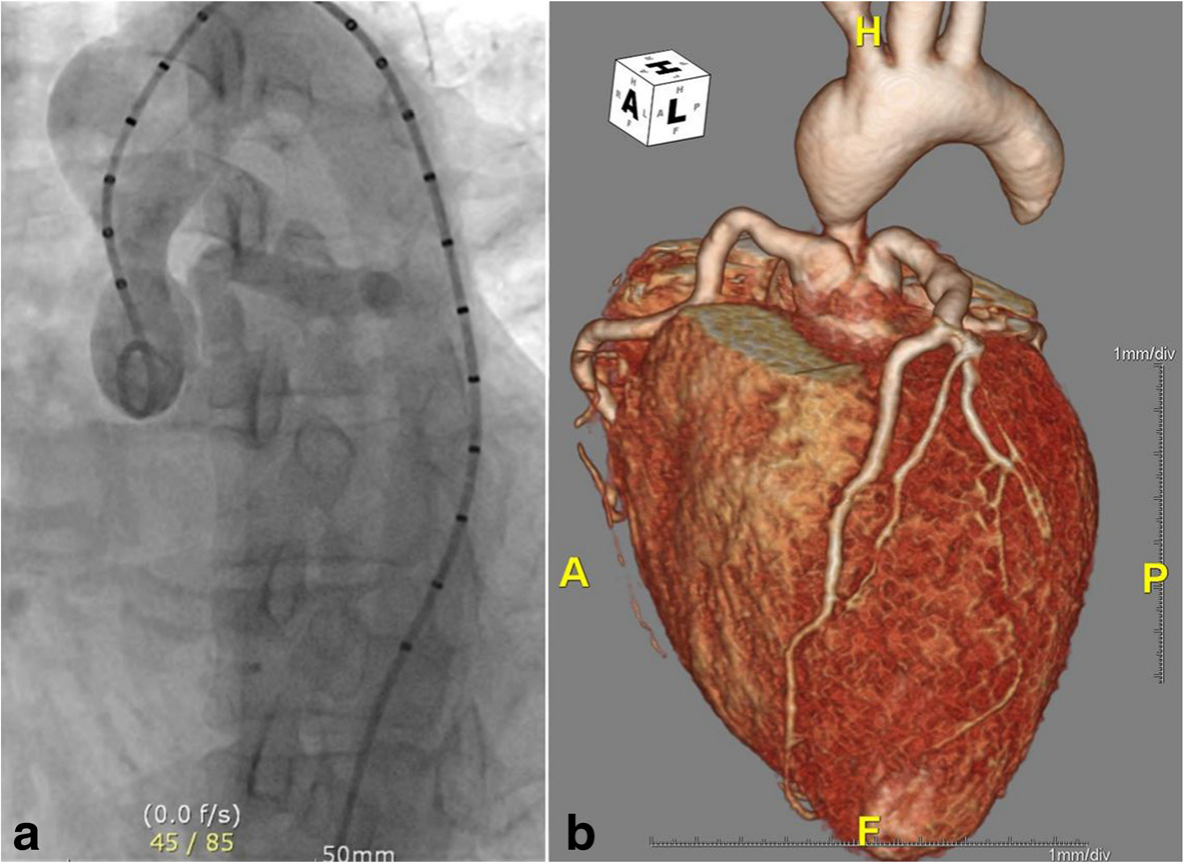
a Aortography showing a focal, hourglass-type supra-valvular aortic stenosis with normal morphology of aortic arch and descending thoracic aorta. b Three-dimensional reconstruction imaging of computed tomography showing the spatial and anatomical relationship of the stenotic aortic root and coronary orifices
